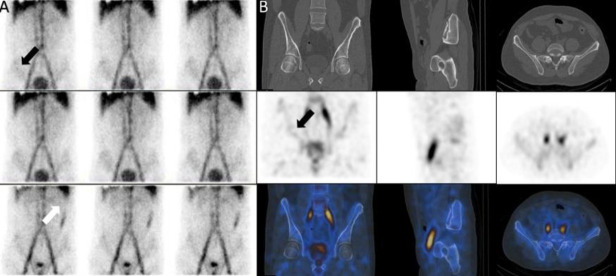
The dynamic scan and SPECT/CT showed activity in bone marrow of iliac alae (black arrows). The scan also showed GIB with source of splenic flexure of colon (white arrow, A)
